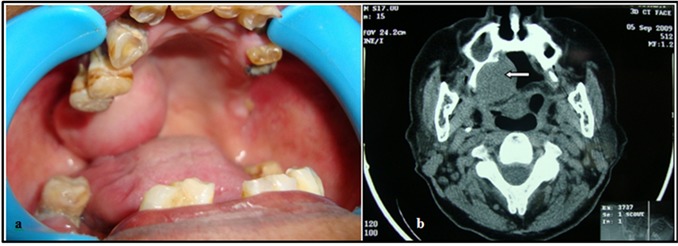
a: Intraoral view shows swelling on the right hard palate  b: CT scan demonstrates soft tissue density mass on the right side of palate (arrow)
